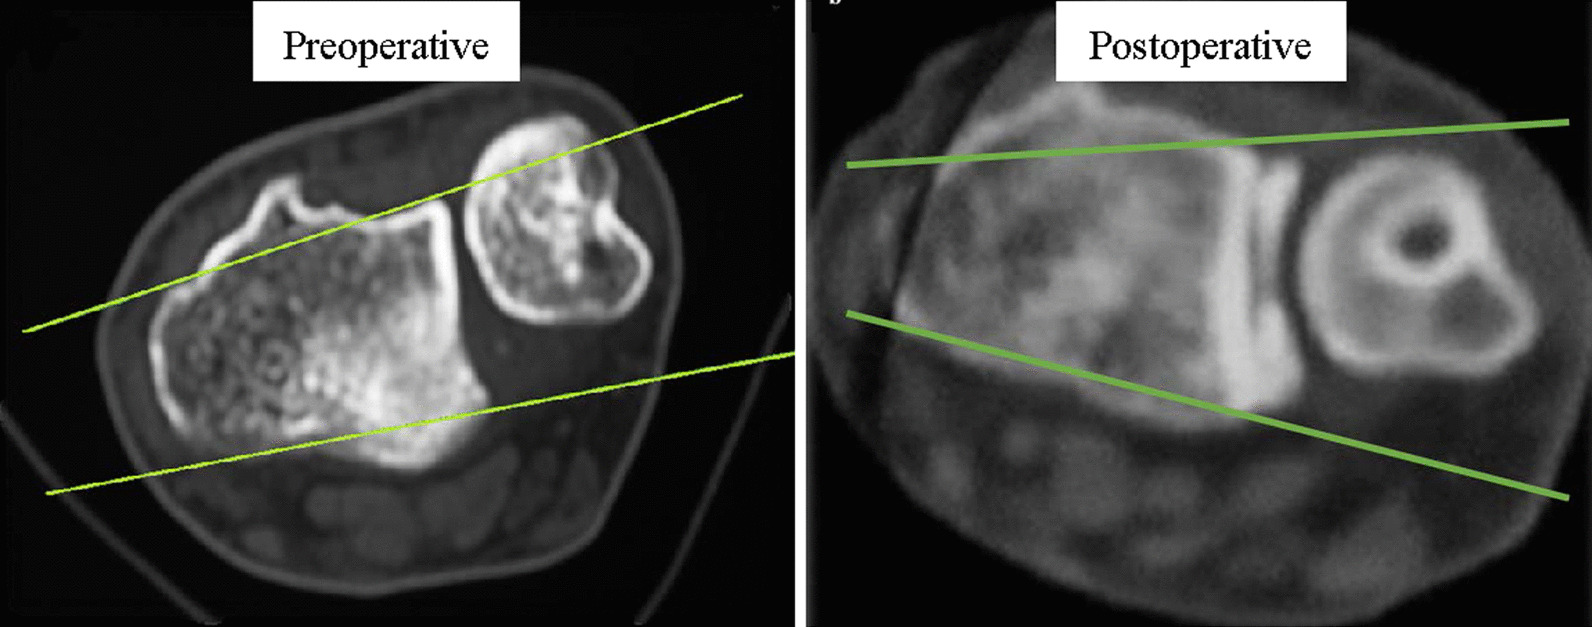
Computed tomography (CT) scan of wrist in neutral position. Preoperative CT scan shows distal radioulnar joint subluxation. Postoperative CT scan shows the ulnar head located between the two tracing lines that define the dorsal and palmar borders of the radius
